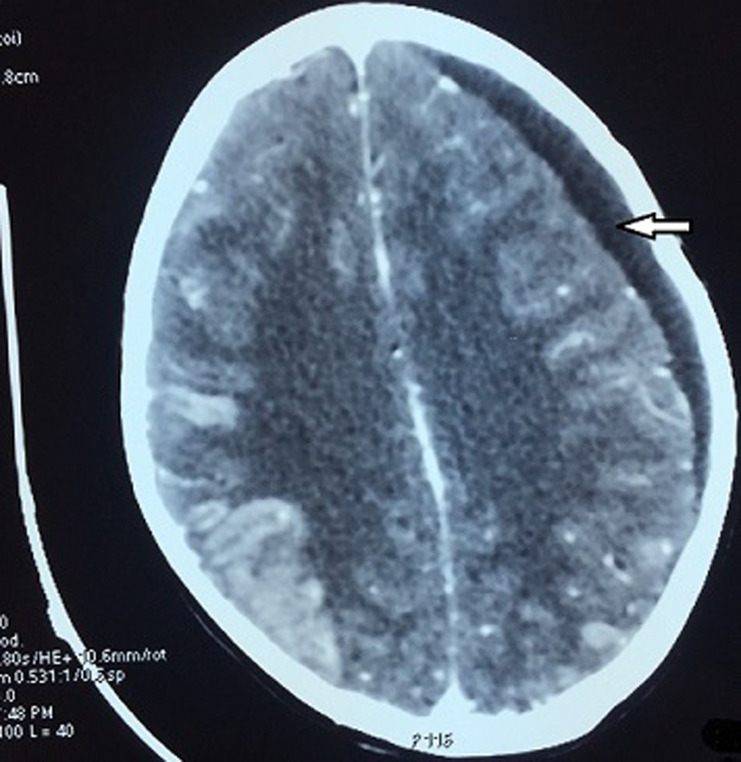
CT of the brain showing subdural chronic hematoma and a serpenginous gyriform enhancement around the contusion in the right parietal and occipital lobes
